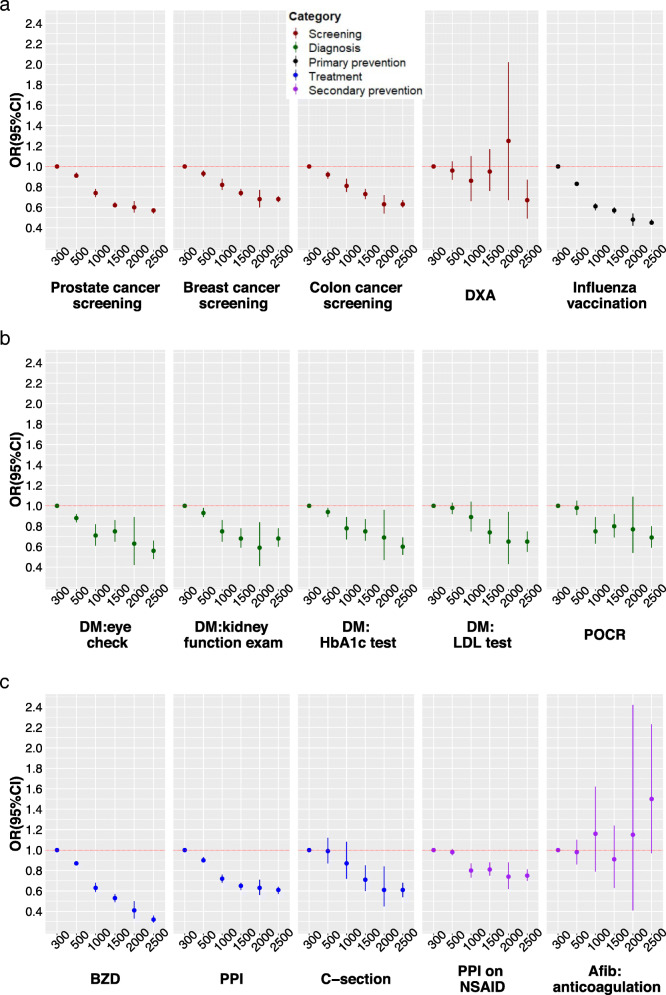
Effects of annual deductible level (Swiss Francs) on healthcare services utilization. OR: odds ratio; CI: confidence interval; DM: diabetes mellitus; DXA: Dual-energy x-ray absorptiometry; HbA1c: glycated hemoglobin; LDL: low-density lipoprotein; TSH: thyroid stimulating hormone; POCR: outpatient preoperative chest radiography; BZD: benzodiazepines; PPI: proton pump inhibitor; C-section: Cesarean section; AMI: acute myocardial infarction; ACE: angiotensin converting enzyme; ARB: angiotensin receptor blocker; P2Y: clopidogrel, prasugrel or ticagrelor; NSAID: nonsteroidal anti-inflammatory drug; PAD: peripheral artery disease; Afib: atrial fibrillation; GKK: Glucocorticoid
